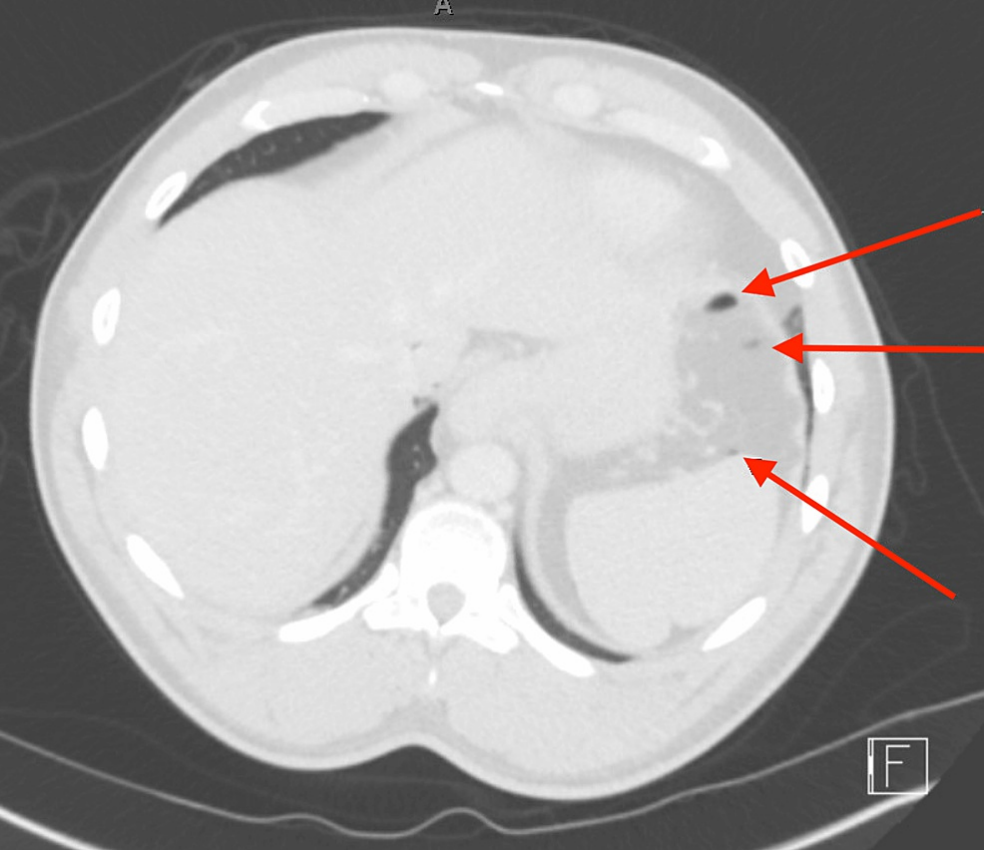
Axial CT image showing punctate air within the abdominal compartment.
